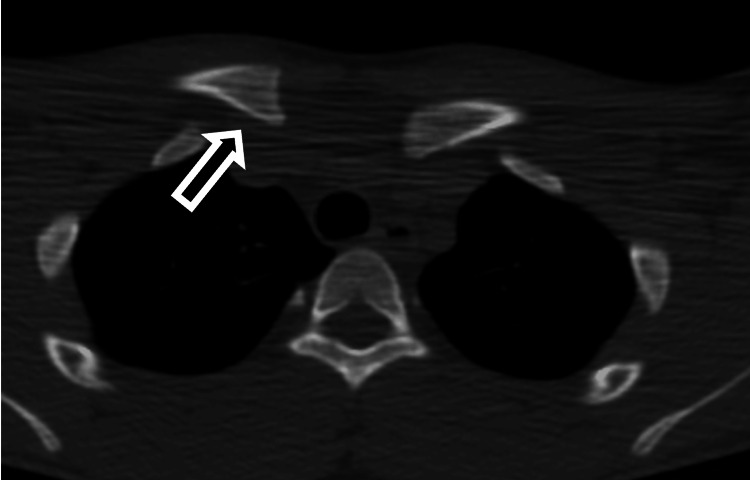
Post-reduction computed tomography scan Axial computed tomography scan of both sternoclavicular joints taken after closed reduction. The medial end of the left clavicle (black arrow) is positioned more anteriorly than the right.
